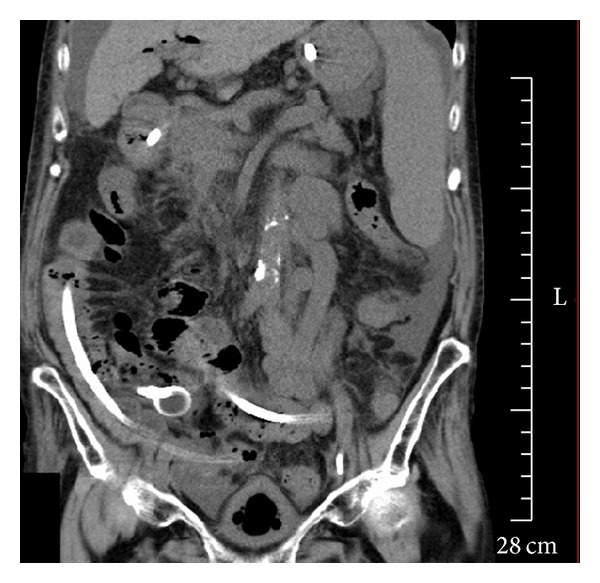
Abdominal CT scan performed immediately after colonoscopy showing the absence of a stone and improved intestinal distension.
